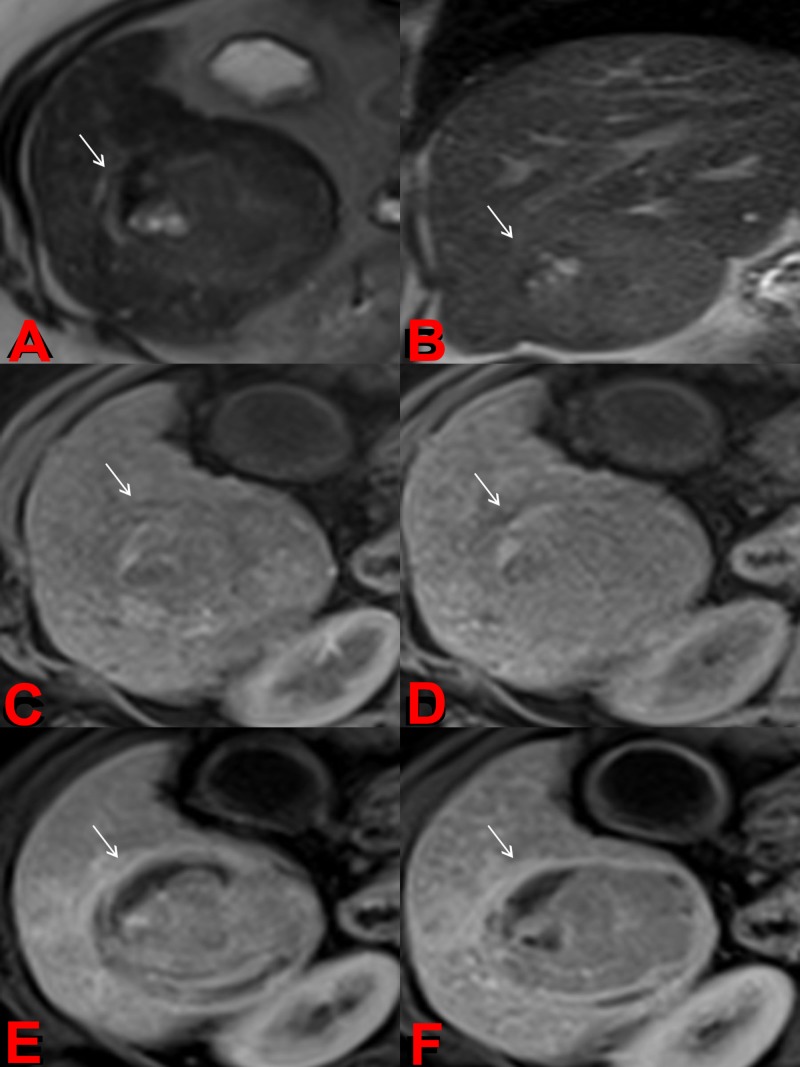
Post-treatment Magnetic Resonance Imaging (MRI) Axial (a) and coronal (b) T2-weighted images demonstrate an overall decrease in signal intensity of treated HCC with interval development of hyperintense foci compatible with cystic necrosis. Precontrast (c), arteral (d), portalvenous (e), and delayed (f) postcontrast T1-weighted images demonstrate lack of tumor enhancement compatible with nonviable tumor and necrosis.
